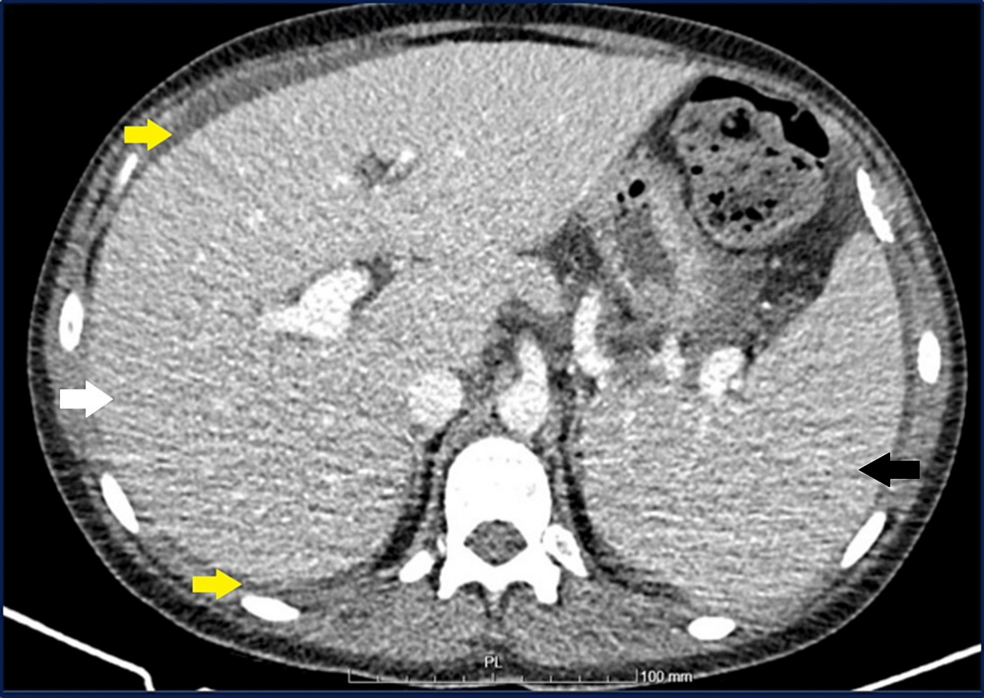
Cross-section of abdominal computed tomography showing hepatomegaly (white arrow), splenomegaly (black arrow), and free peritoneal effusion (yellow arrow).
